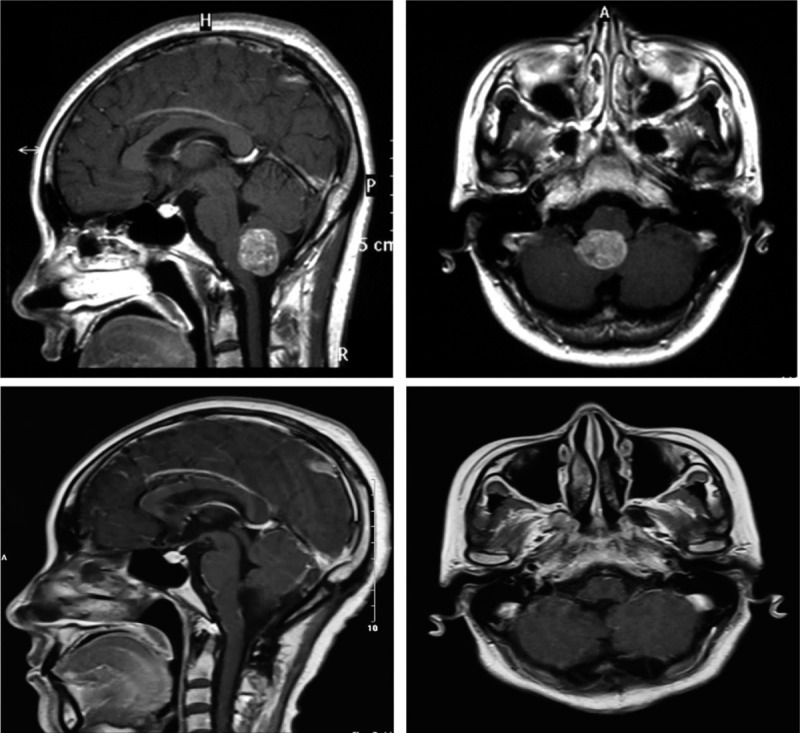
Preoperative cranial magnetic resonance suggested a circular abnormal signal at the posterior margin of the foramen magnum, with clear boundaries and a size of about 2.5 cm × 1.9 cm × 2.6 cm. After enhanced scanning, the signal was significantly enhanced, and the dorsal medulla oblongata was compressed. Postoperative MRI on the second day after surgery suggested complete resection of the tumor.
